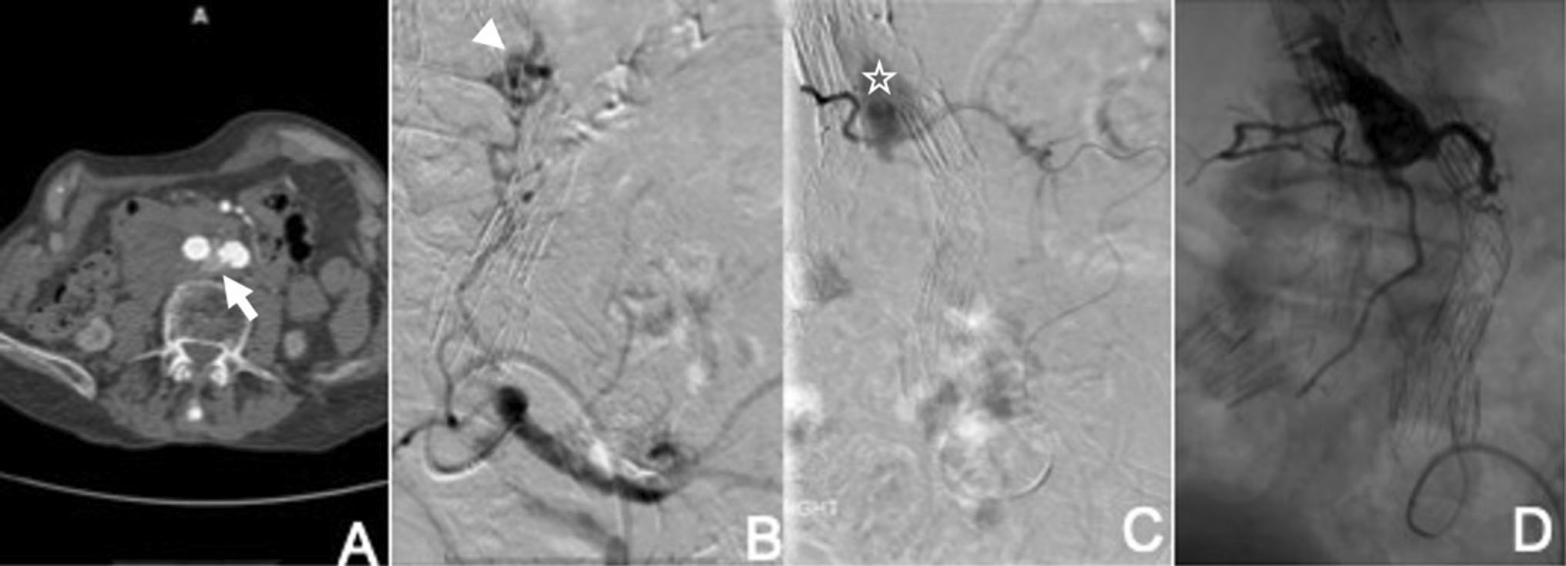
Transarterial embolisation of type 2 endoleak arising from iliolumbar artery. A Axial CT image shows endoleak arising from a left lumbar artery (arrow). B Angiogram following catheterisation of the left internal iliac artery shows filling of the endoleak cavity (nidus) by the left lumbar artery (arrowhead). C Angiogram following selective microcatheter catheterisation of the endoleak cavity through the tortuous iliolumbar artery shows endoleak cavity (asterix) and several exit vessels. D Complete embolisation of endoleak cavity and exiting branches using Onyx
